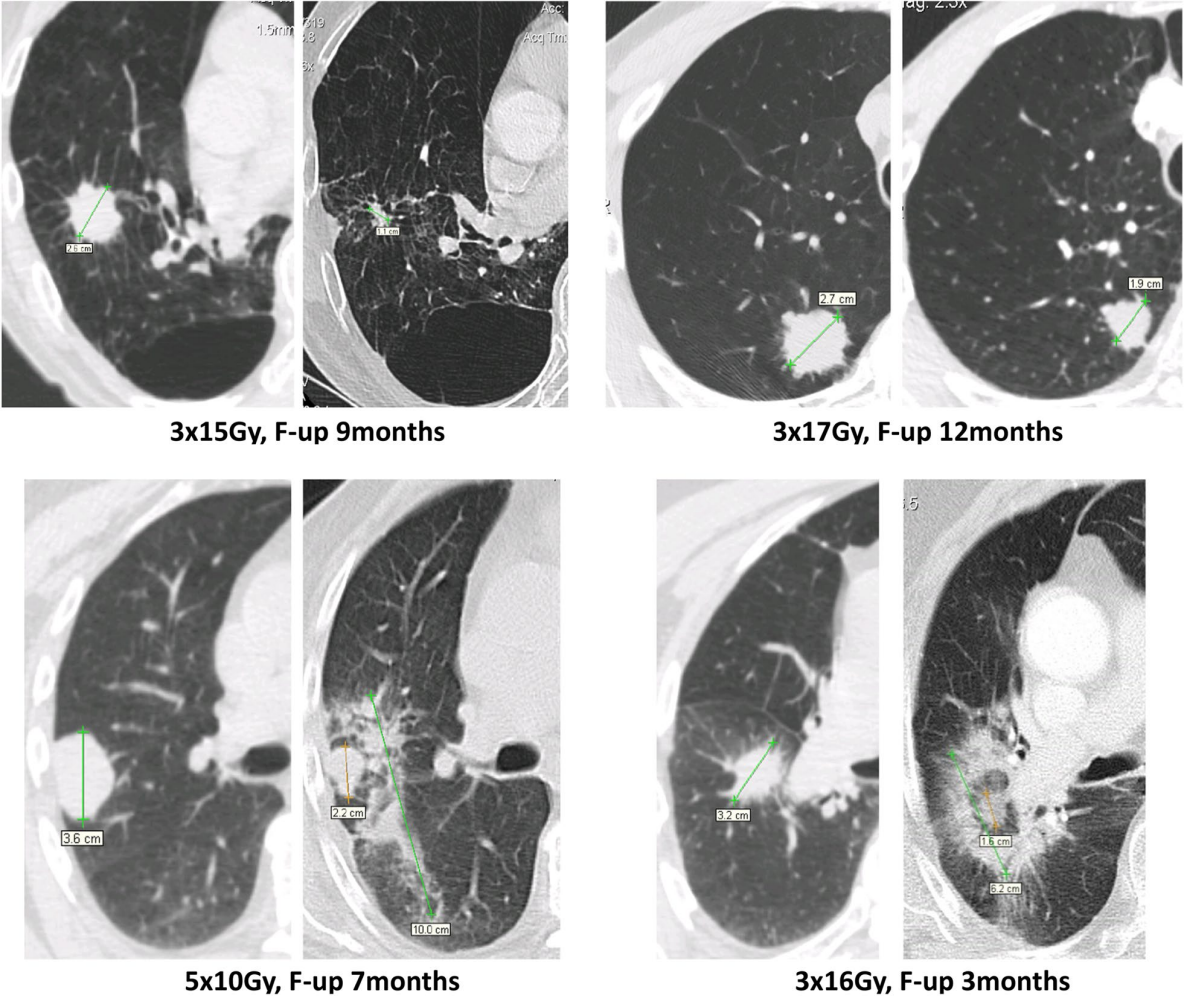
Representative chest CT images of patients who did not (upper row) or did (lower row) develop local lung injury induced by robotic stereotactic body radiation therapy of early-stage non-small cell lung cancer
